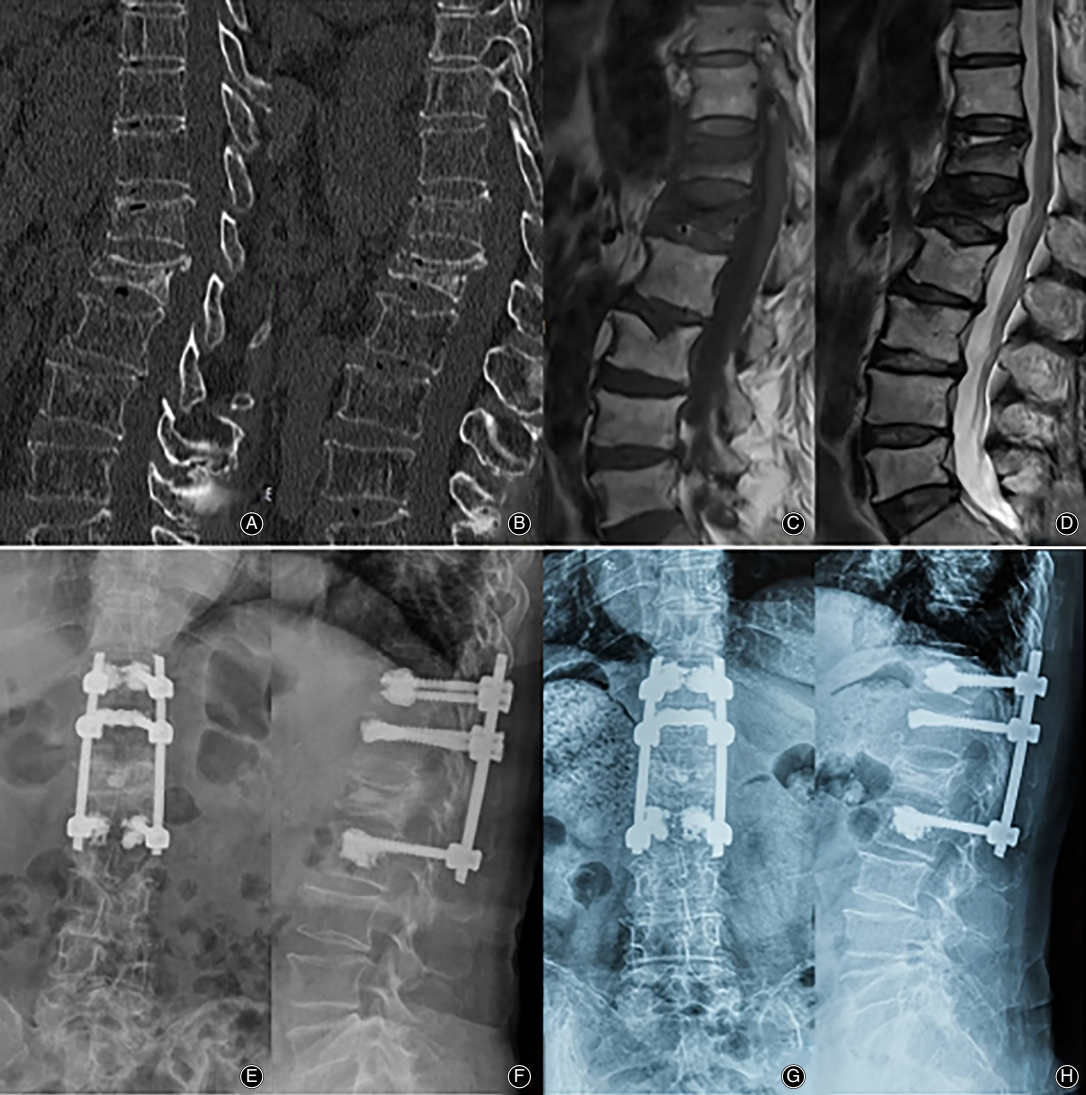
A 76‐year‐old female patient who underwent SSF for T_12_ and L_1_ Kummell disease. (A‐B) Preoperative CT scan showing T_12_ and L_1_ fracture with IVC, and MRI showing IVC on the T1(C) and T2‐weighted (D) images. Postoperative (E and F) and final follow‐up (G and H) X‐rays revealing good internal fixation position and satisfactory radiographic results.
